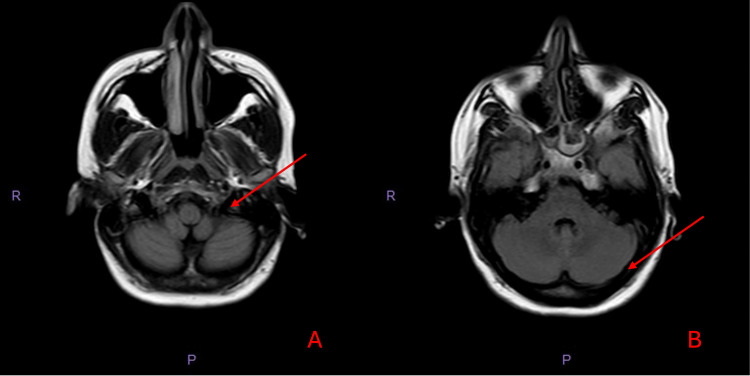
Day 7 MRI head A: Normal appearances of the left sigmoid sinus on FLAIR MRI (arrow). B: Normal appearances of the left transverse sinus on FLAIR MRI (arrow). MRI: magnetic resonance imaging; FLAIR: fluid-attenuated inversion recovery
